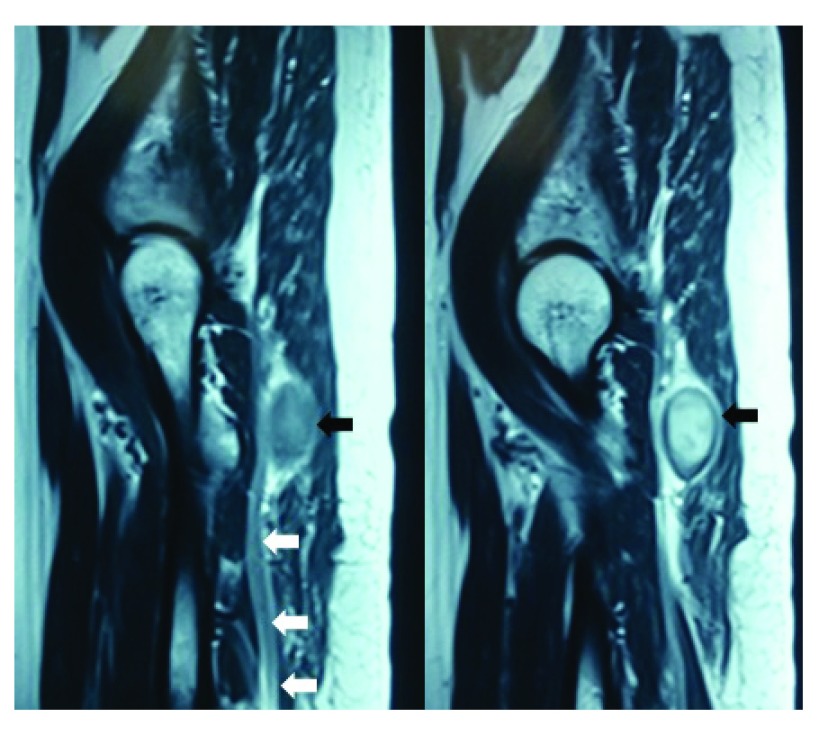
MRI scan revealing the unusual location of the tumor with a hypo-intense rim along the course of the sciatic nerve.
